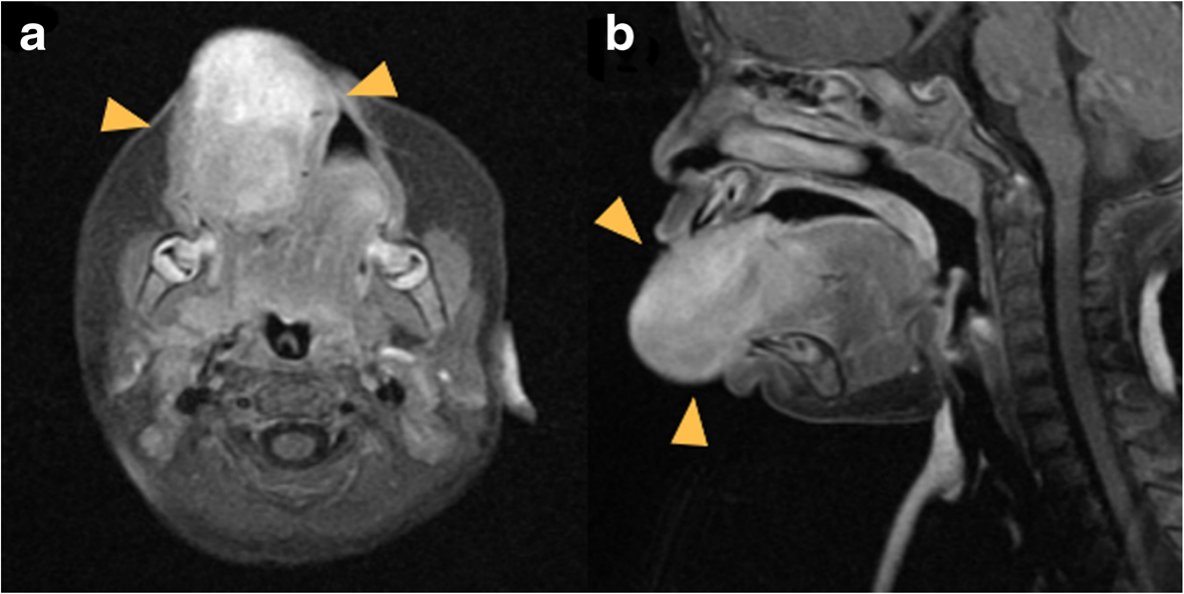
a Magnetic resonance imaging (MRI) showing huge mass with contrast enhancement in the right side. b MRI of saggital plane did not show the posibility of infiltration into the root of the tongue. Arrows indicate the areas of tumor with contrast enhancement
